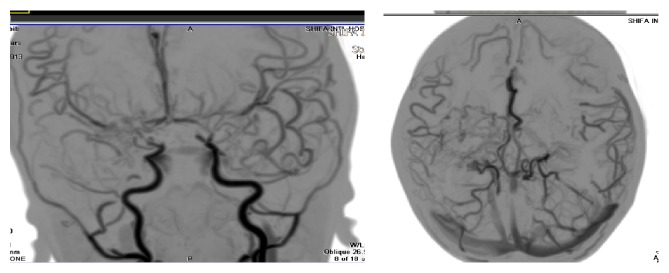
Preoperative CTA head showing stenosis of supraclinoid parts of bilateral ICA.
